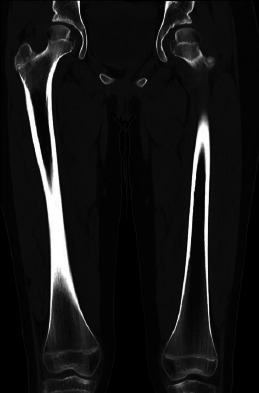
Coronal reformatted CT images of both femurs in a 12-year-old female.CT imaging of the femurs demonstrates bilateral genu valgum. No evidence of physeal abnormality, bone dysplasia, or other structural abnormalities was identified.
